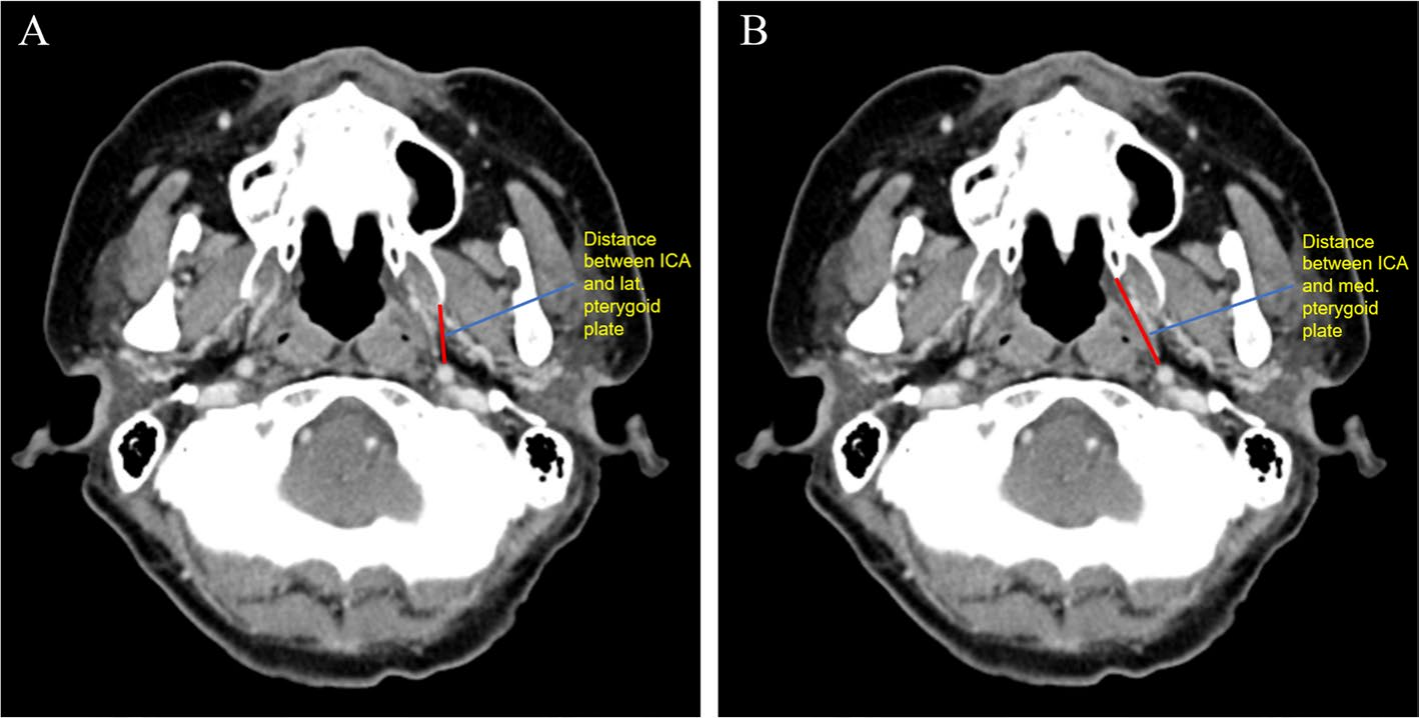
The distance from the internal carotid artery (ICA) to the (A) lateral and (B) medial pterygoid plates was measured on the axial CT image at the level of nasal floor
